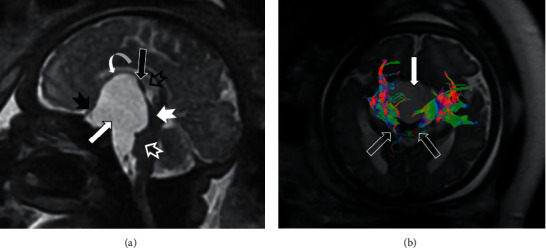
Arachnoid cyst associated with persistence of Liliequist membrane. Gestational age: 36 weeks and 2 days. (a) Sagittal SSFSE T2. Large-sized arachnoid cyst (solid white arrow) anterior to the mesencephalon extending into the prepontine cistern and suprasellar region is shown. The cyst produces a slight mass effect of the protuberance (empty white arrowhead) and an important mass effect on the mesencephalon (solid white arrowhead), hypothalamic region (empty black arrowhead), mammillary bodies (solid black arrow), the floor of the third ventricle (curved white arrow), and optic chiasm (solid black arrowhead). (b) Diffusion tensor with axial tractography image. The cyst (solid white arrow) extends towards the interpeduncular cistern of the mesencephalon and displaces the corticospinal tracts in a posterolateral direction (empty white arrows). Fetal MRI provides additional information because the real extent of the lesion is better evaluated than with ultrasound.
